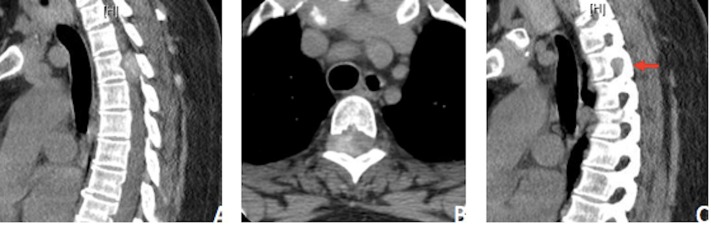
Preoperative Thoracic Spine CT A. Sagittal CT thoracic spine without contrast, demonstrating areas of patchy hyperdensity within the lesion suggestive of calcifications. B, C. Axial and sagittal CT thoracic spine without contrast showing bony remodeling and widening of the right T3-4 neural foramen.
